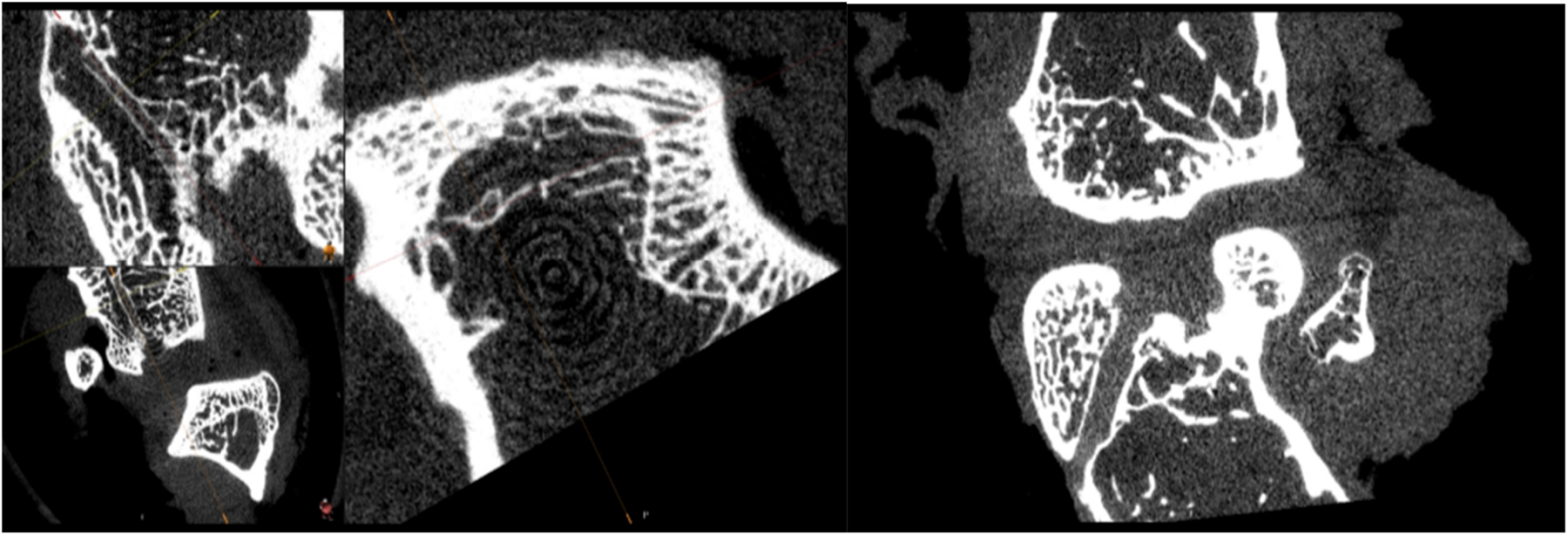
Sections of the computed tomography (CT) image measuring tunnel widening. The tunnel diameter was measured on the sagittal view in regions of interest (proximal, middle and distal) in the femoral and tibial tunnels.
